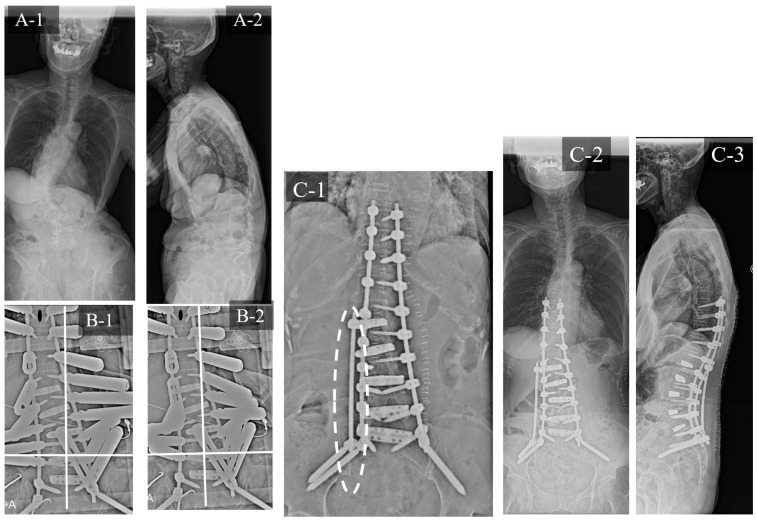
Case study 3 (RR and KR). (A-1) Preoperative standing whole spine radiograph (AP). (A-2) Preoperative standing lateral whole spine radiograph. (B-1) Intraoperative radiograph before RR. (B-2) Intraoperative radiograph after RR. (C-1) Intraoperative radiograph after KR. (C-2) Postoperative standing whole spine radiograph (AP). (C-3) Postoperative standing lateral whole spine radiograph. RR: rod rotation, KR: kickstand rod technique.
